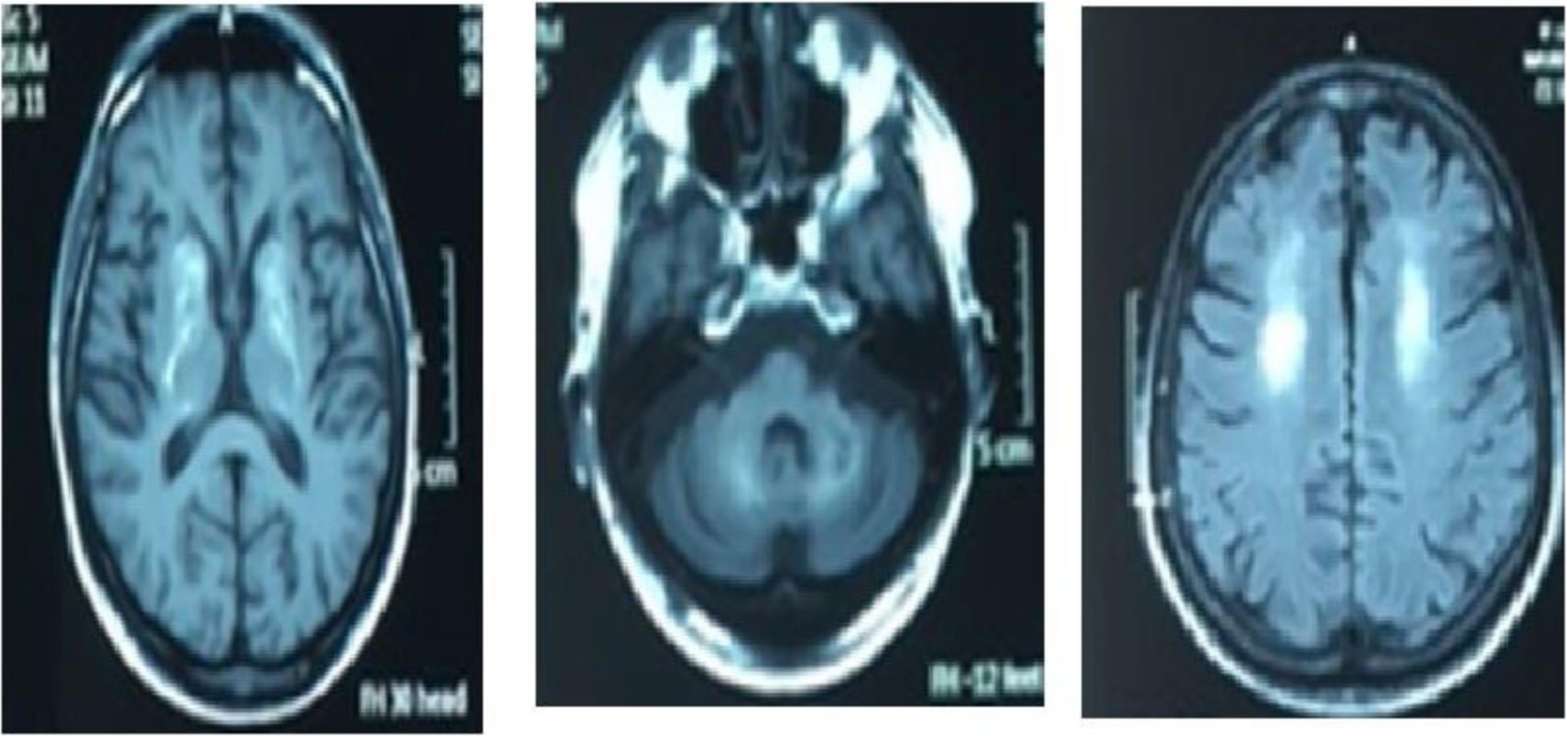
Shows the Magnetic resonance imaging (MRI) scan of the brain revealing bilateral basal ganglia thalamic and dentate ganglia calcification
